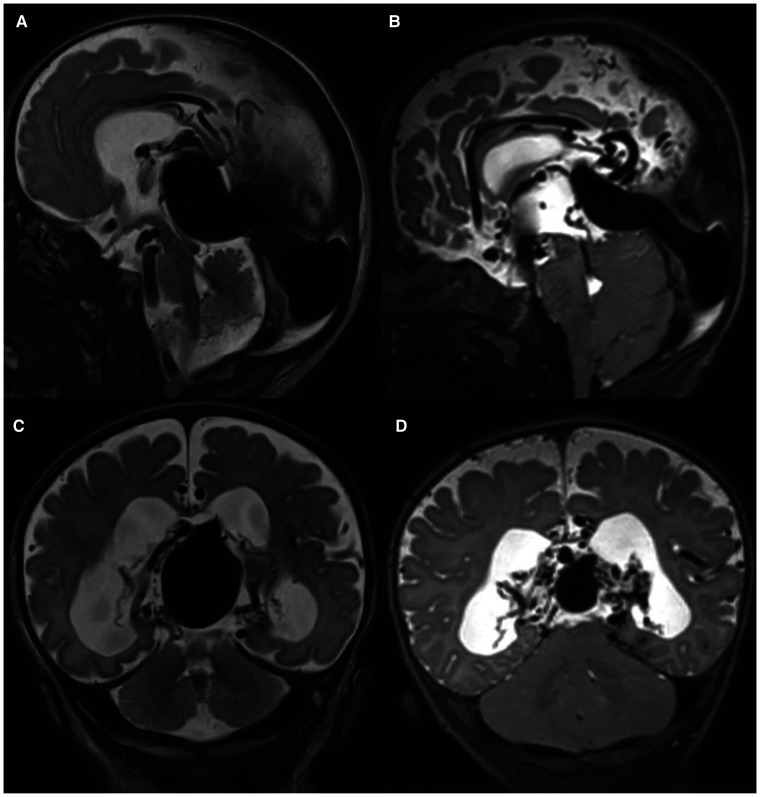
Brain MRI features: T2w sagittal (A,B) and coronal (C,D) images show the presence of VGAM at birth (A,C) and after embolization (B,D) in patient P5.
